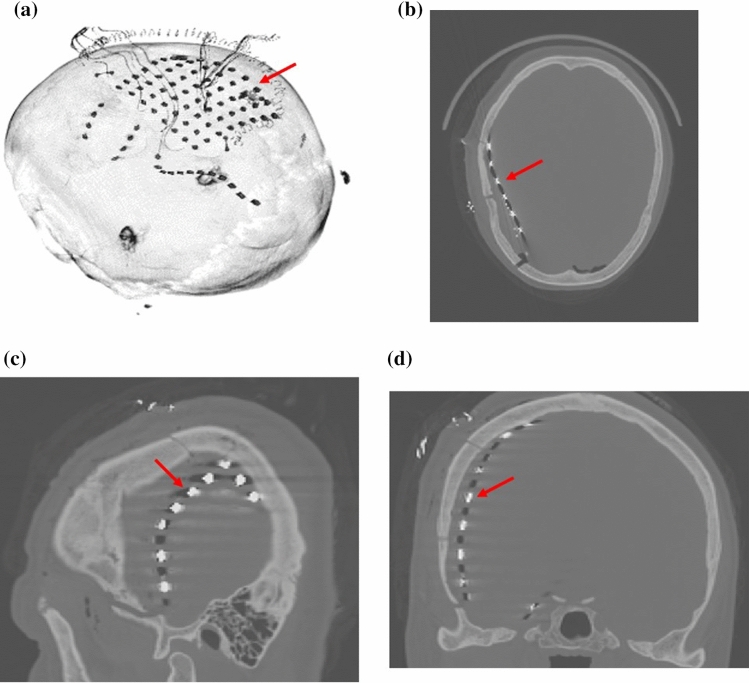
CT volume of Neuromed patient #15: a 3D rendering, b–d axial, sagittal and coronal cut planes. The red arrows point to electrodes. In the 3D rendering both an 8 × 8 grid electrodes and some strip electrodes are clearly visible. Cranium cuts are clearly recognizable in the axial view
